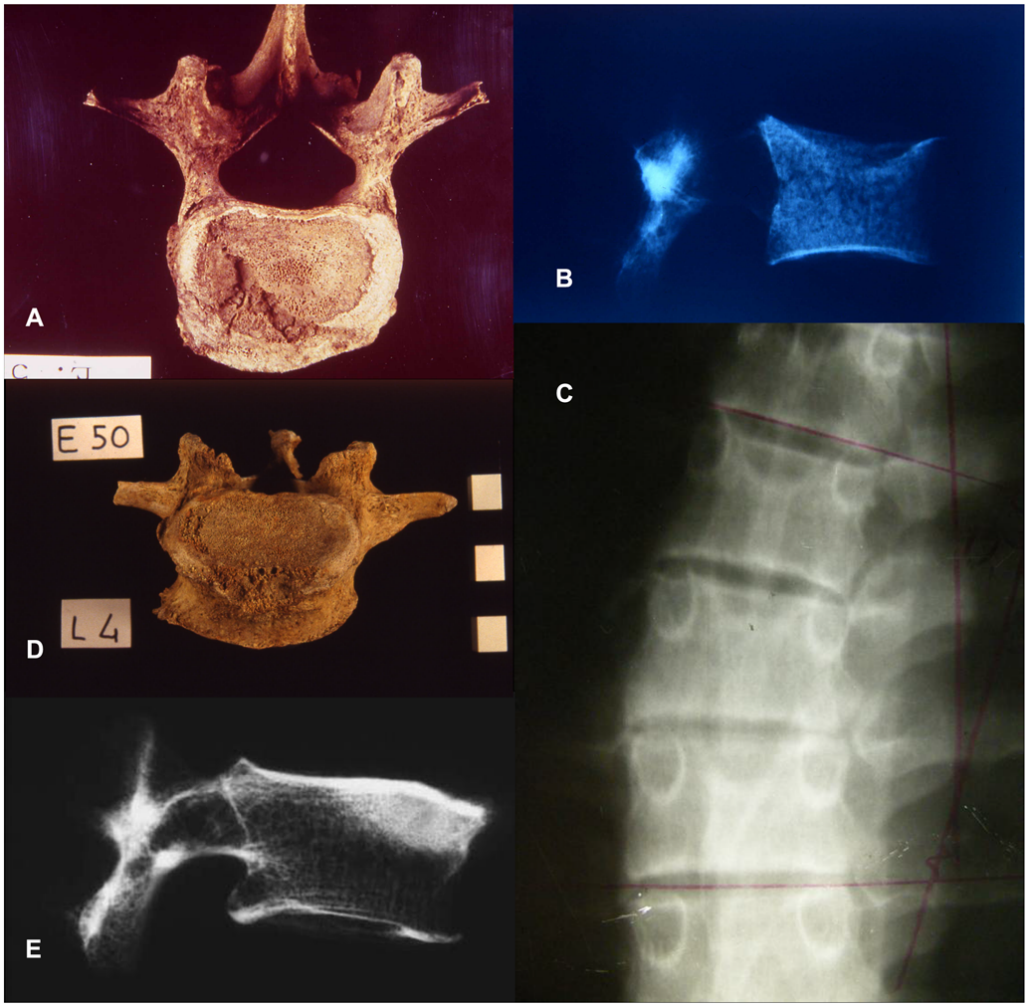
The lytic lesions on Stw's 431 L4 and L5 were compared with ancient modern human lumbar vertebrae affected by brucellosis and tuberculosis, and with Scheuermann's disease in a living young male. Modern human L4 affected by tuberculosis: macroscopic (A) and radiographic picture (B) [18] (Courtesy of Capasso; adult female E58, Herculaneum, 79 AD, University Museum of Chieti). Scheuermann's disease in the vertebral column of a living 18 years old male: Radiographic analysis (C) (Courtesy of Zipfel; School of Anatomical Sciences at the University of the Witwatersrand). Modern human L4 affected by brucellosis: macroscopic (D) and radiographic picture (E) [18] (Courtesy of Capasso; adult female E50, Herculaneum, 79 AD, University Museum of Chieti).
